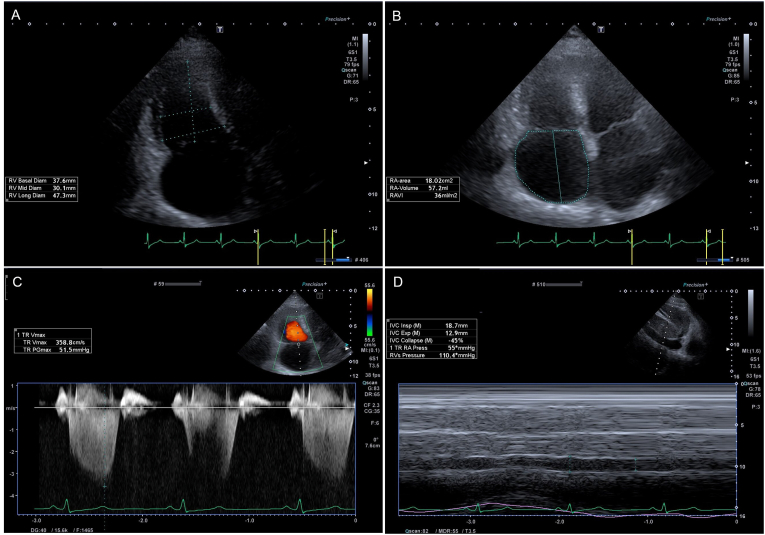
Echocardiographic Evaluation of Right Heart Chambers and Pulmonary Hemodynamics Transthoracic echocardiographic assessment. (A) Apical four-chamber view focused on the right ventricle (RV) demonstrating basal (37.6 mm) and mid-cavity (30.1 mm) diameters within normal limits according to ASE guidelines. (B) Quantification of the right atrium (RA) showing a right atrial volume index (RAVI) of 36 mL/m^2^, consistent with moderate chamber dilation. (C) Continuous-wave Doppler recording of the tricuspid regurgitation jet demonstrating a peak velocity of 3.58 m/s and a peak pressure gradient of 51.5 mmHg. (D) M-mode assessment of the inferior vena cava (IVC) showing a diameter of 18.7 mm with 45% inspiratory collapse, resulting in an estimated right ventricular systolic pressure (RVSP) of 110.4 mmHg.
